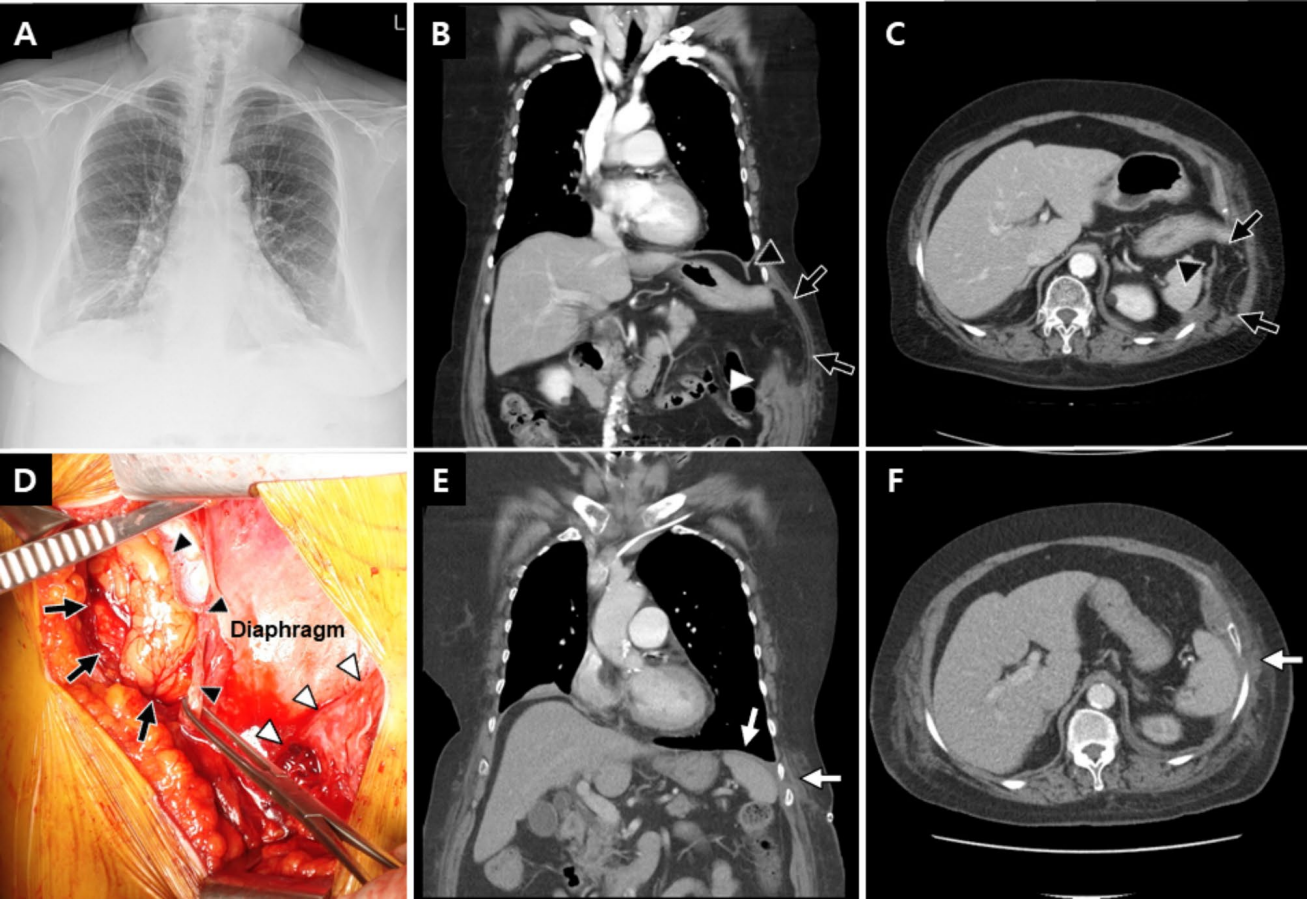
Chest X-ray and thoracoabdominal computed tomography (A, B and C) before surgery showing herniation of the lung and intra-abdominal organs into the thoracic and abdominal walls (black arrows) through the ruptured diaphragm (black arrowhead), as well as the intercostal and abdominal muscles (white arrowhead); the operative findings (D) demonstrating herniated intra-abdominal organs (black arrows) through the ruptured diaphragm (black arrowheads) and parietal pleura (white arrowheads); the thoracoabdominal computed tomography (E, F) after surgery revealing reduced herniation, an intact diaphragm, and repair of the left ninth intercostal space (white arrows)
